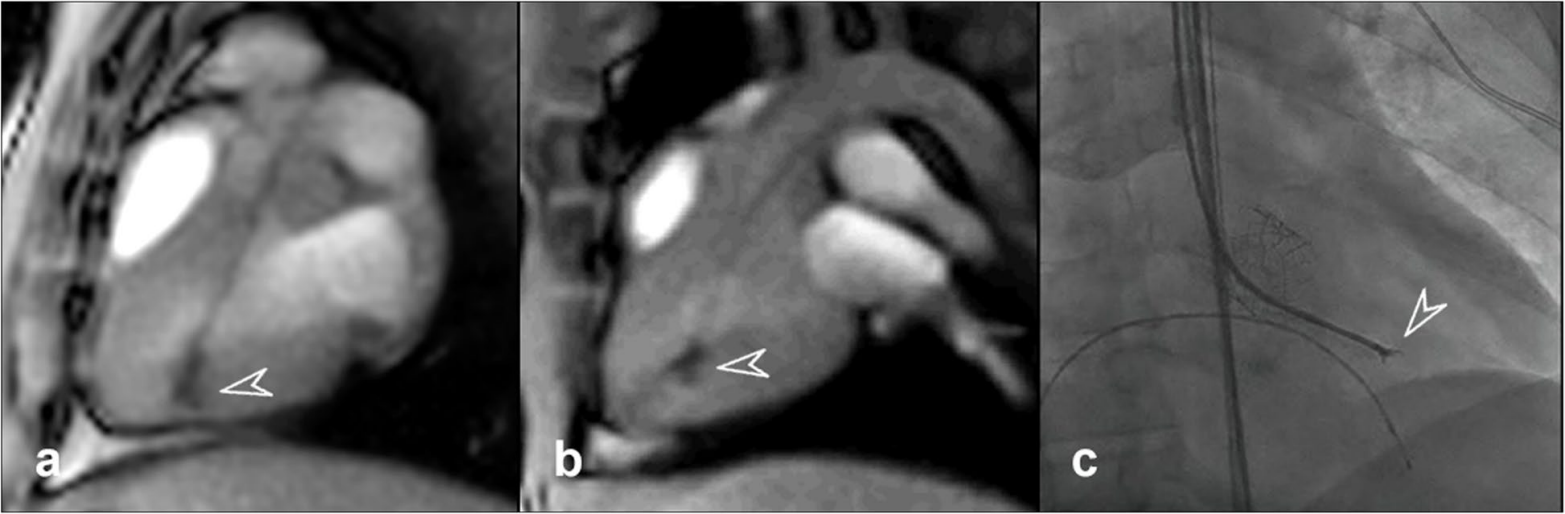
Real-time magnetic resonance imaging-based endomyocardial biopsy procedure depicting the “open” state during the *in vivo* procedure with B1 (a) and B2 (b), featuring visible cardiac walls, major vessels and the timing of the cardiac cycle. X-ray fluorography-based standard procedure in a patient showing an ‘open’ state control forceps (c)
